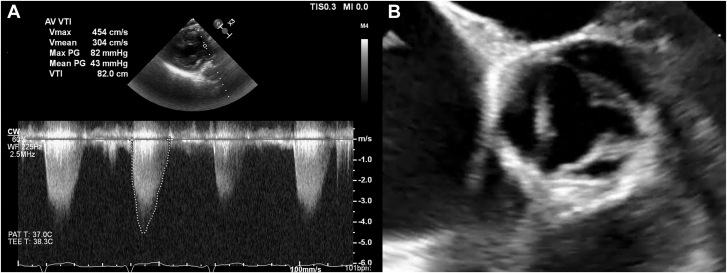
(A) Gradient across aortic valve and LVOT on transthoracic echocardiogram. (B) Normal aortic valve opening.
